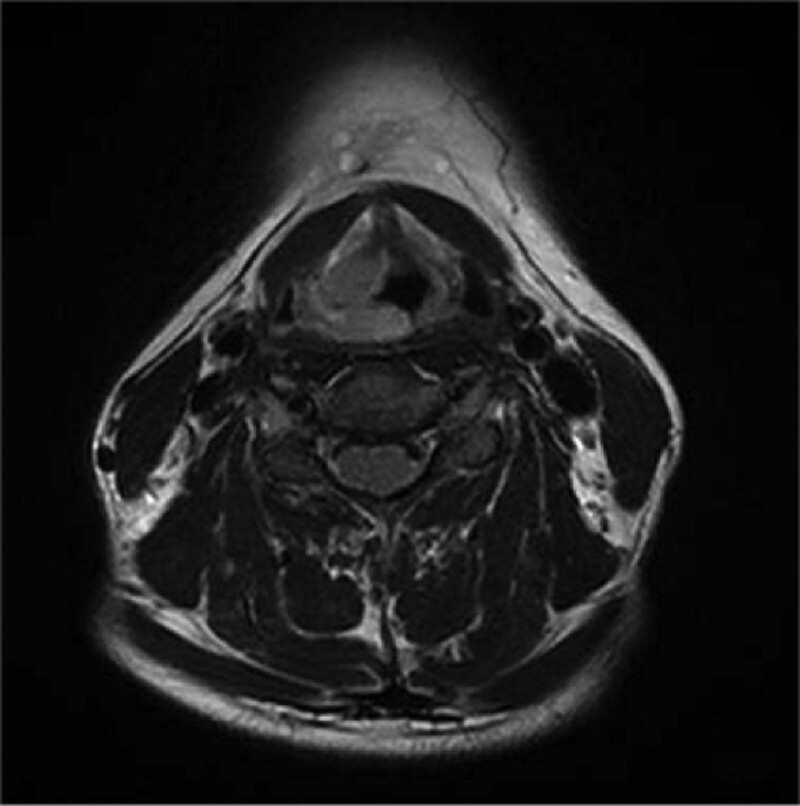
Head and Neck MRI: Head and Neck MRI revealed that the contrast-enhancing soft tissue is located in the posterior wall of the nasopharynx, the right wall of the oropharynx, the right arytenoepiglottic fold, and the right ventricular zone thickened irregularly with axial section. MRI = magnetic resonance imaging.
